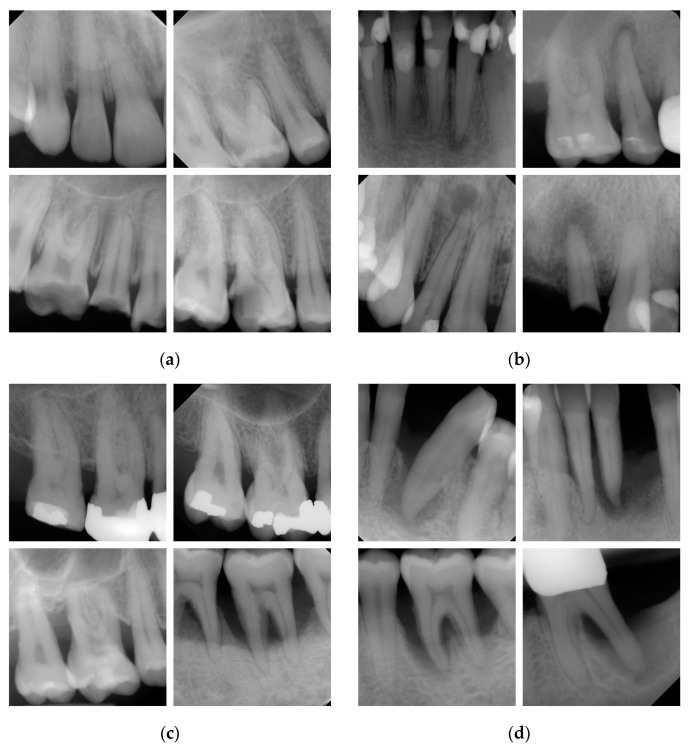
Examples of periapical radiographs for the four criteria: different stages of (a) caries, (b) periapical lesions (c) periodontal bone loss (PBL) and (d) endo-perio lesions.
